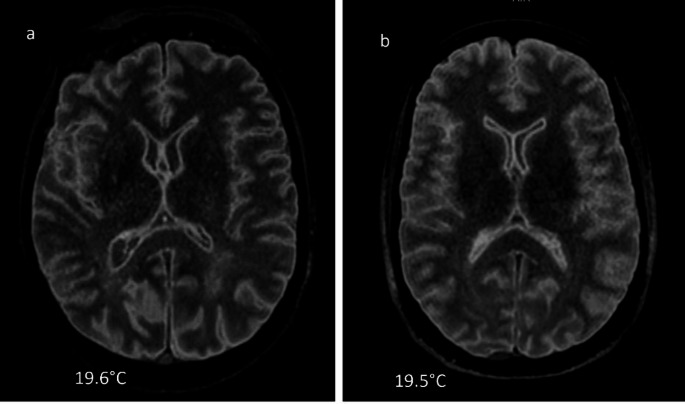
Axial reconstruction of a 3D sagittal cerebral FLAIR acquisition with a TI at 1660 ms: a) & b) two corpses at 19.5 °C with similar contrast image
